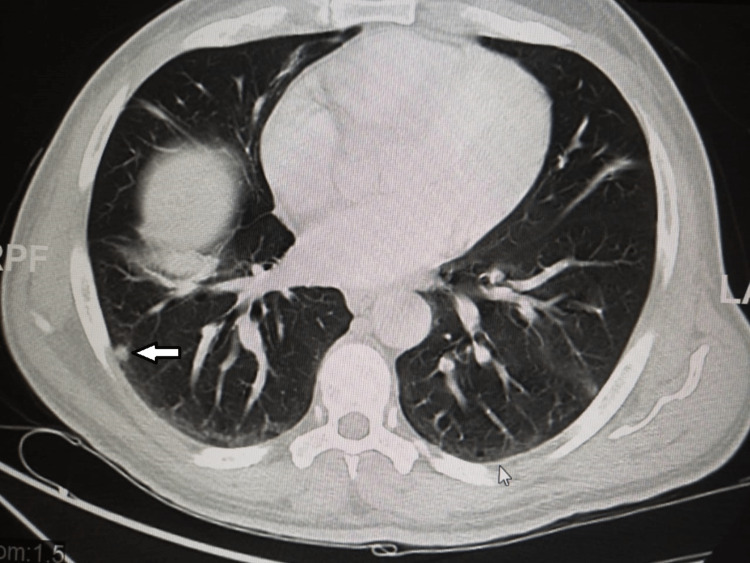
A CT scan of the chest The scan shows a small sub-pleural nodule-like consolidation (white arrow).
